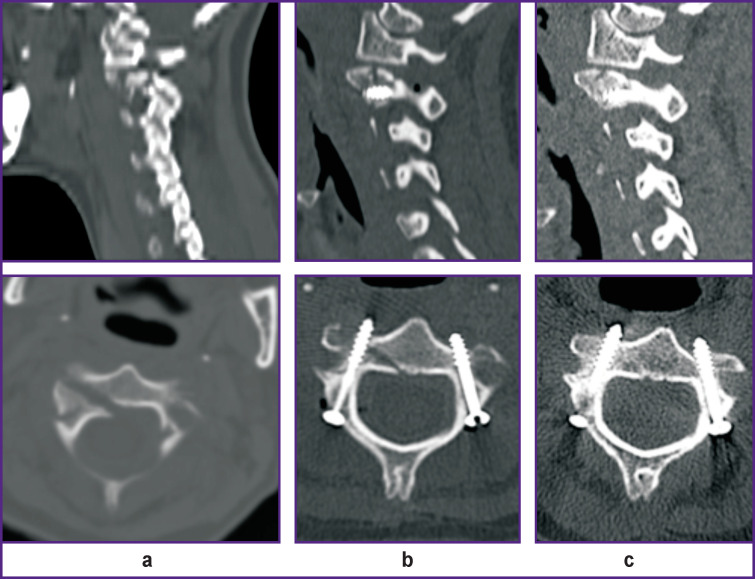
CT scans of the 22-year-old female patient with traumatic spondylolisthesis of the C_2_ vertebra: (а) preoperative sagittal and axial scans, fracture is visualized, diastasis between fragments on the right >3.5 mm; (b) 1 day after the operation, transpedicular screws are visualized, no marked malposition (>2 mm) is observed; (c) 6 months after the operation, fracture consolidation is noted
